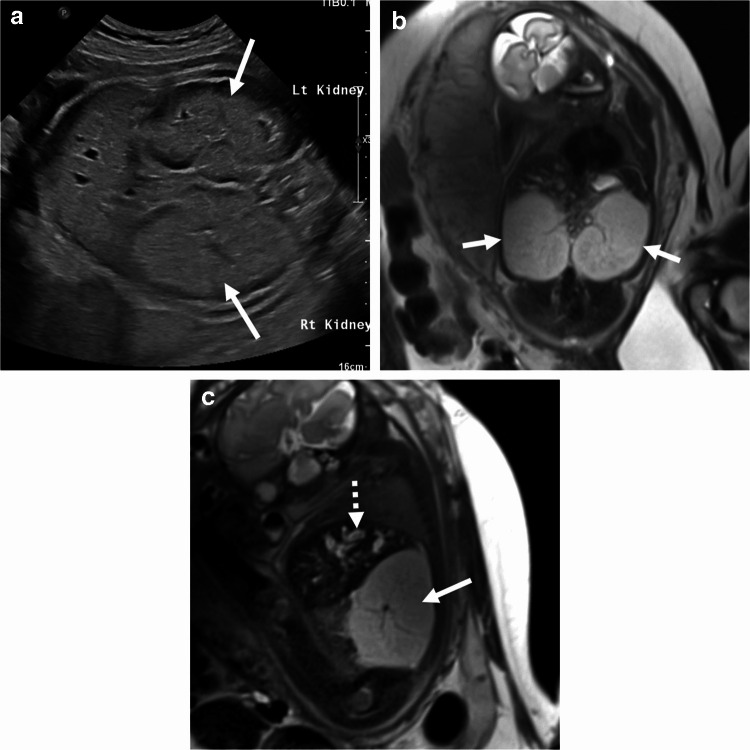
a Coronal fetal US of a 30-week gestation age male fetus with autosomal recessive polycystic kidney disease (ARPKD) showing bilateral enlarged kidneys with diffuse increased echogenicity (arrows). b Coronal Single-Shot Fast Spin Echo (SSFSE) MRI images obtained the same day showing massively enlarged bilateral kidneys (arrows) with diffuse increased T2 signal without discrete cysts. Small appearing chest and hypoplastic lungs are also noted as part of Potter’s sequence. c Sagittal SSFSE MRI showing enlarged, T2- hyperintense kidney (arrow). Caroli’s disease is also present as evidenced by cystic dilation of multiple intrahepatic bile ducts with central dot sign (dotted arrow)
